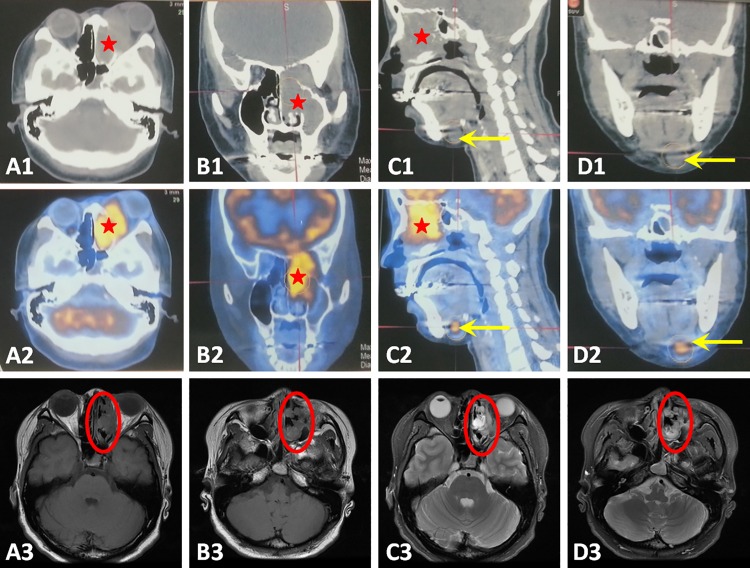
CT scans, PET scans and MRI findings of the tumor and metastatic cervical lymph nodes. The tumor was seen in the left nasal cavity, maxillary and ethmoidal sinus on CT scans (A1, B1and C1, red stars) and PET scans (A2, B2 and C2, red stars). MRI T1-weighted image showed a low-intense mass (A3 and B3, red circles) while T2-weighted image showed a relatively high-intense mass (C3 and D3, red circles). Metastatic cervical lymph nodes can been seen on CT scans (C1 and D1, yellow arrows) but more prominent on the PET scans (C2 and D2, yellow arrows).
